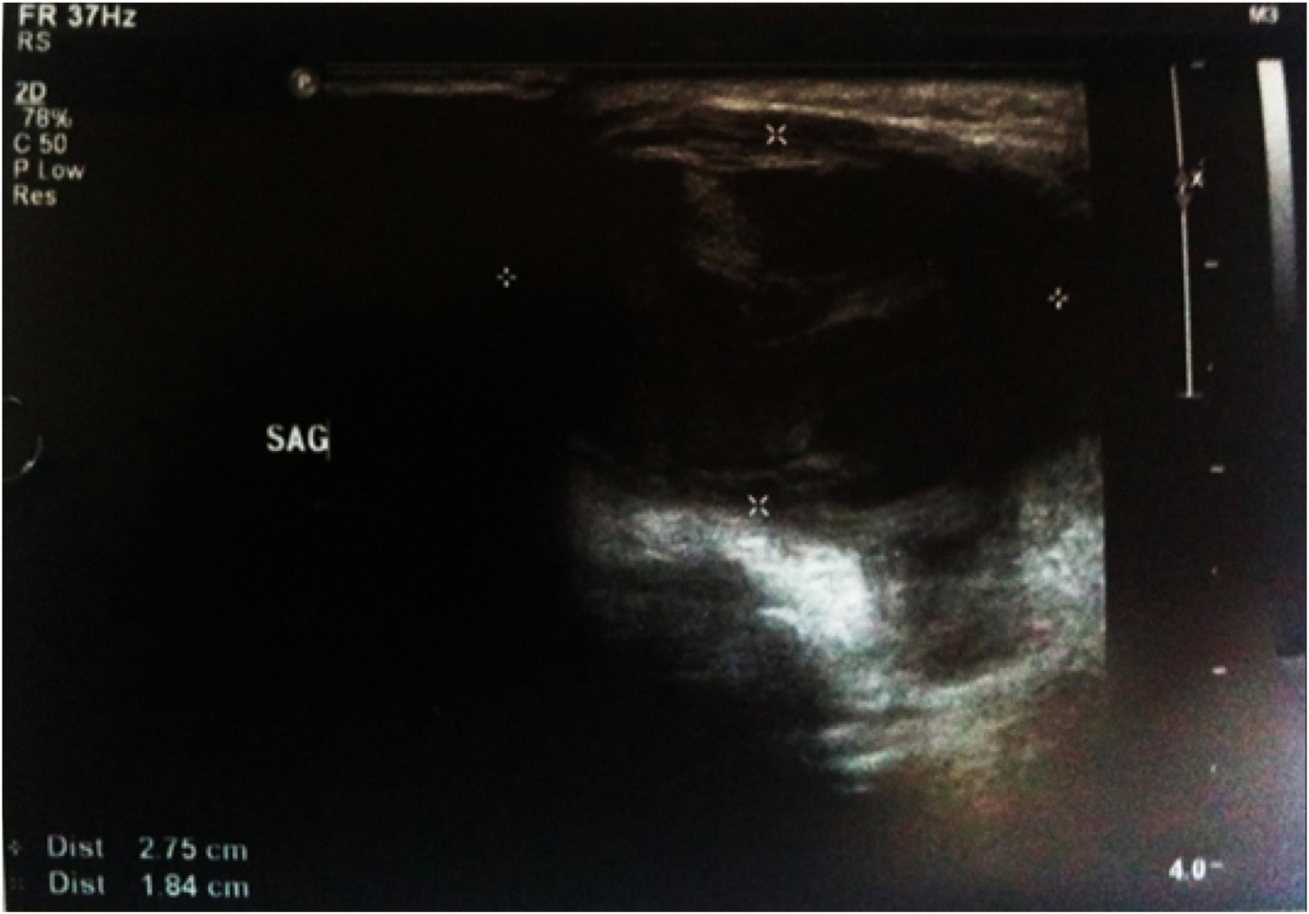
Doppler USG view of the mass.
